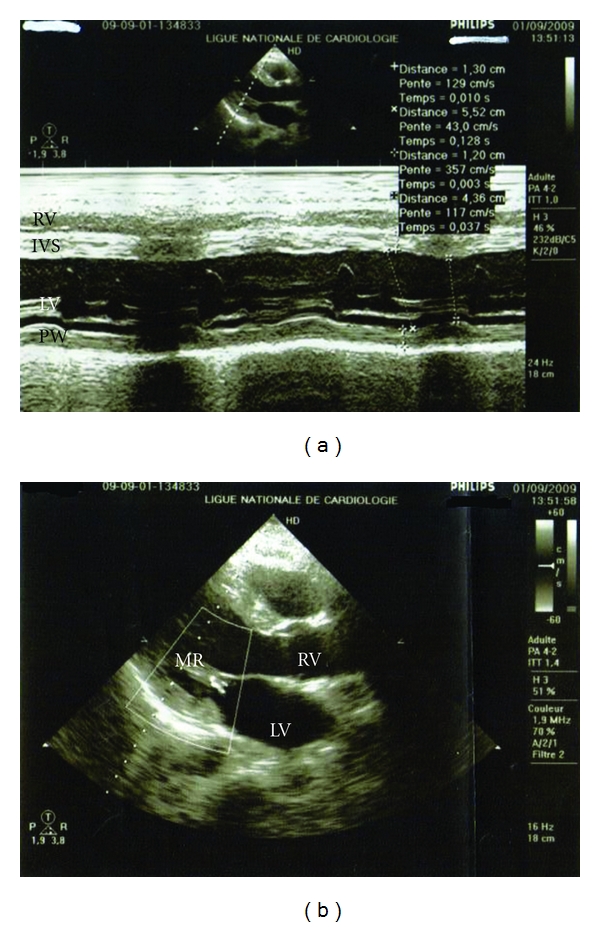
(a) Echocardiographically parasternal long axis depicted improvement of ventricle function. First echocardiogram obtained four days after admission showed LVEF of 48% (calculated by Simpson method). Hypokinesis of the septum and parietal wall of the left ventricle. (b) Second echocardiogram taken 24 days after BWS envenomation depicted good evolution of mitral regurgitation becomes grade I. LVEF was 50%.
